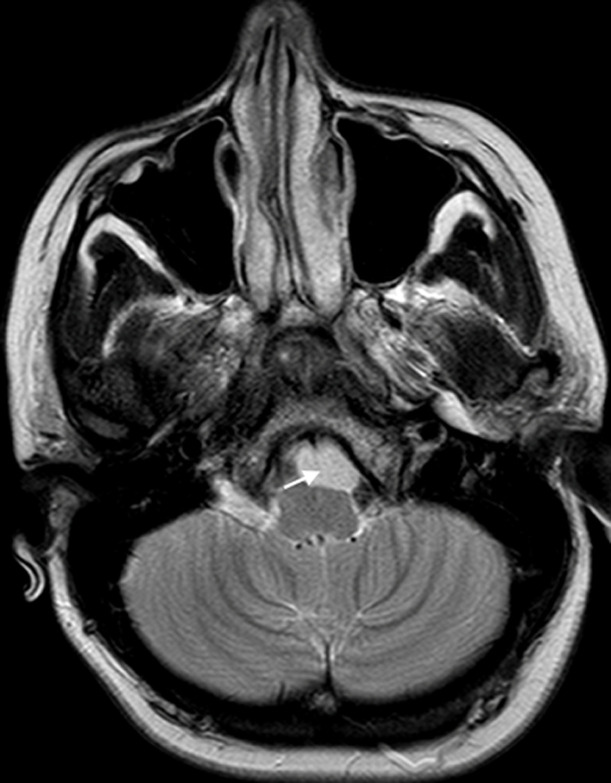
T2 axial MRI showing the lesion posterior to the clivus, with a brainsterm compression
